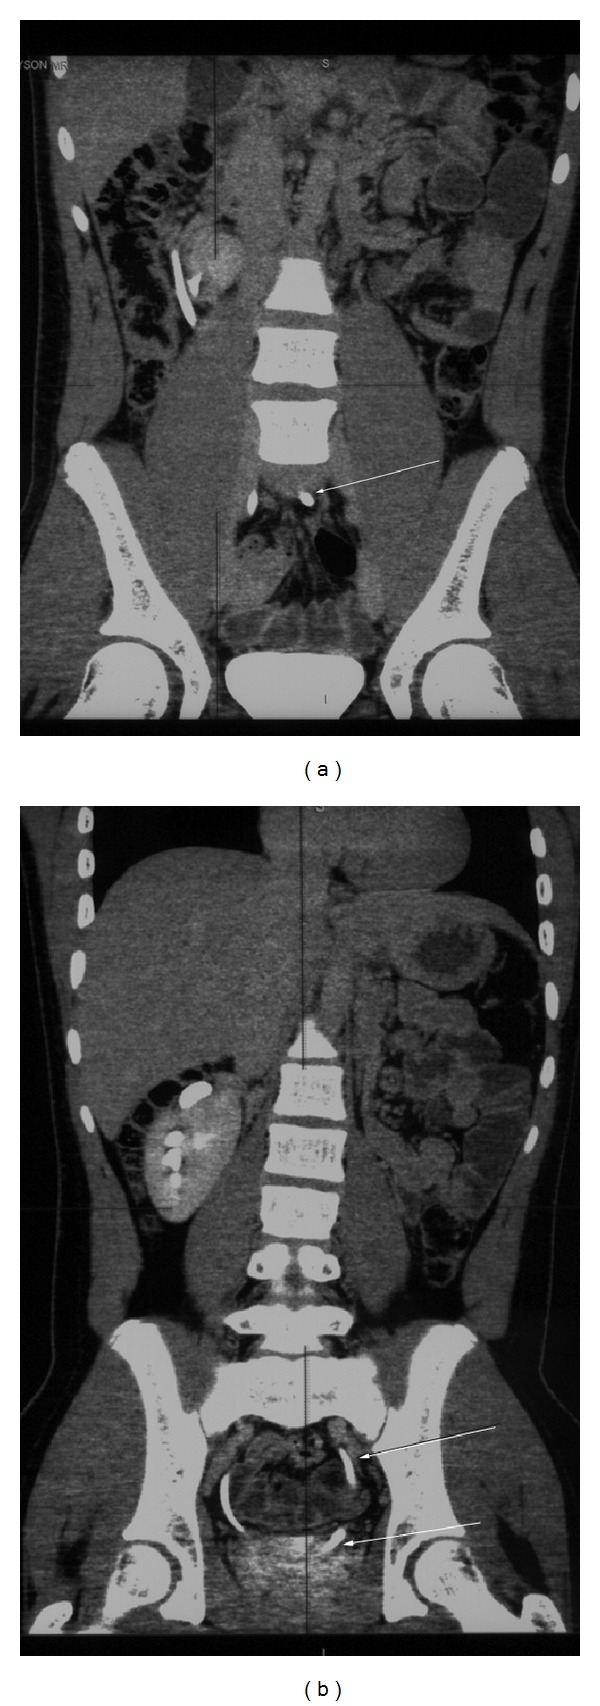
16-year-old adolescent male with left-to-right crossed renal ectopia. Coronal CT scan with intravenous contrast-delayed phase demonstrates the left ectopic ureter crossing midline (white arrow in (a)), to insert onto left side bladder (white arrows in (b)).
